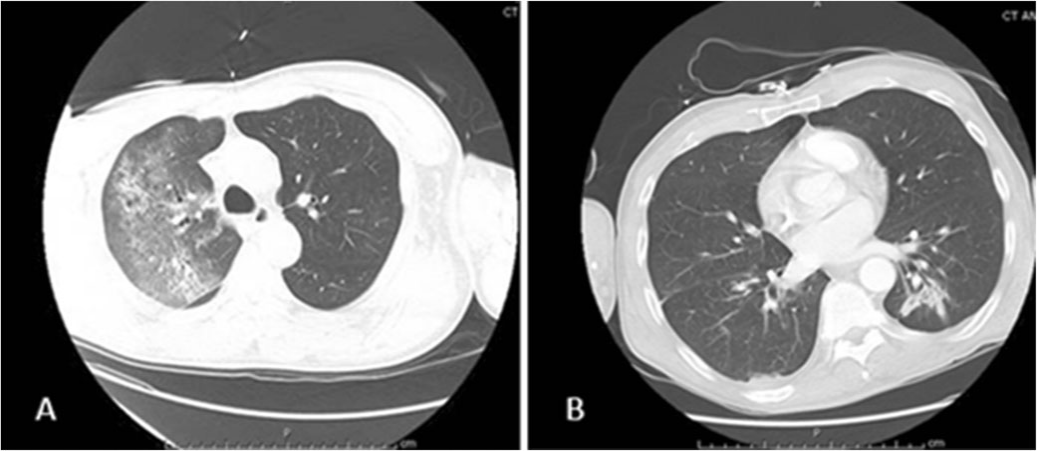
Computed tomography of the chest. A. Patchy opacities inthe right lung. B. Resolution of the opacities after intravenous Amphotericin B.
